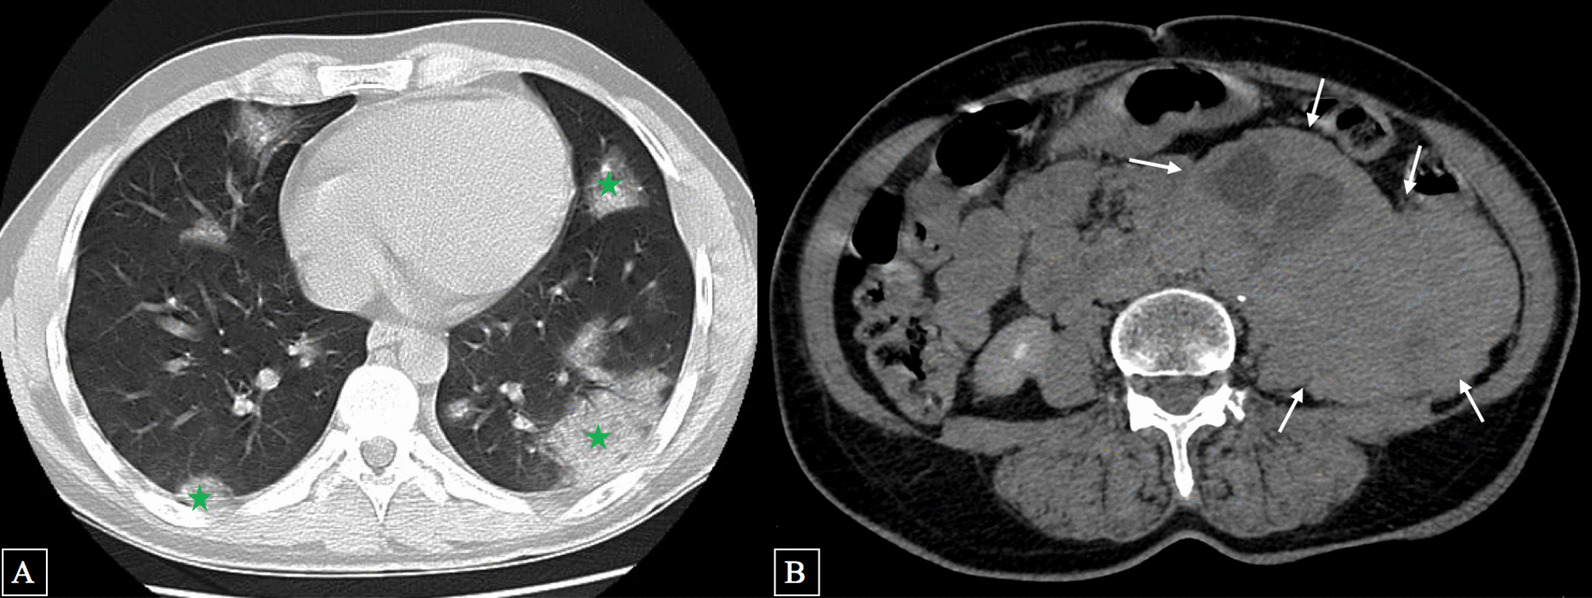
Computed tomography (CT) scan in pulmonary (A) and mediastinal (B) windows revealing ground-glass opacities related to COVID-19 infection (
